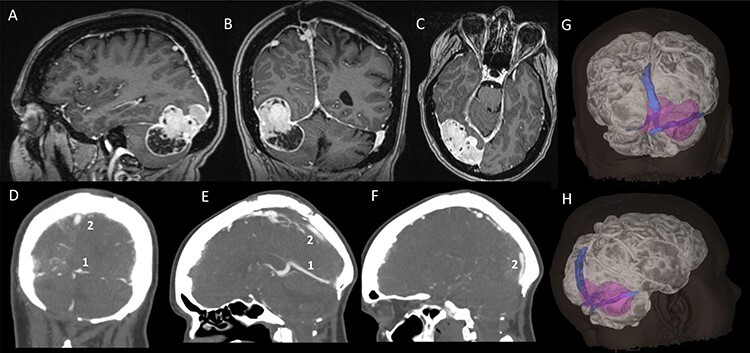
Pre-operative imaging; (A–C) sagittal, coronal and axial T1-weighted MRI images, with gadolinium demonstrating a supra-infratentorial peritorcular tumour; (D–F) CT venogram showing the patency of the straight sinus (number 1) and the superior sagittal sinus (number 2); (G and H) 3D model of the tumour, brain and dural venous sinuses generated using both the MRI images and CT venogram.
